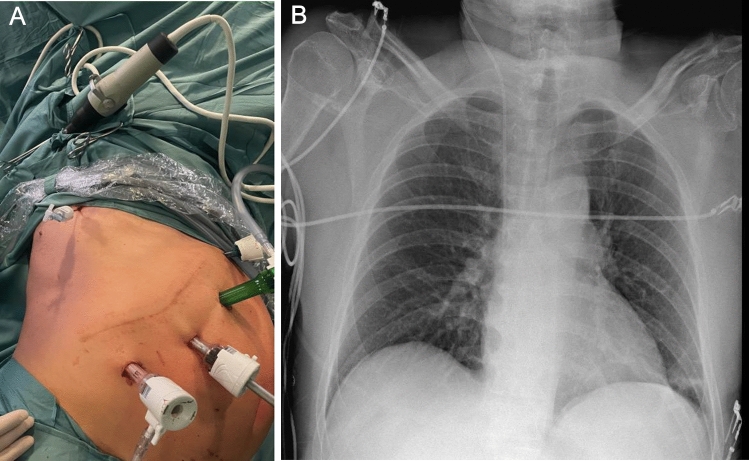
A Placement of the Veress needle between the 7th and 8th intercostal spaces along the midaxillary line. B 18-h postoperative chest X-ray revealing no evidence of residual pneumothorax
